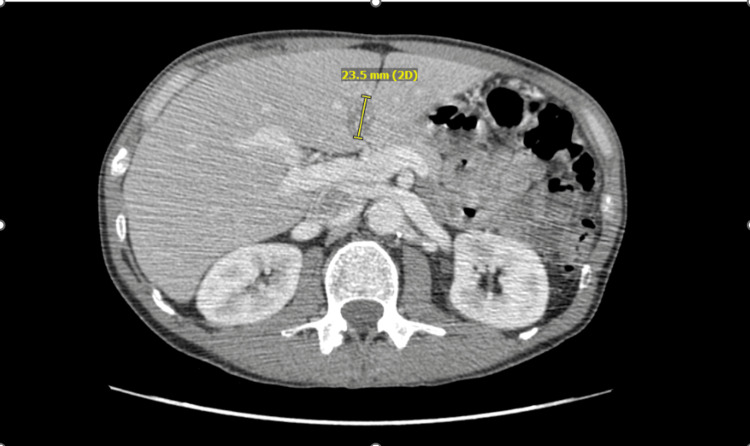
Initial CT abdomen with liver metastasis seen, 06/4/6021
